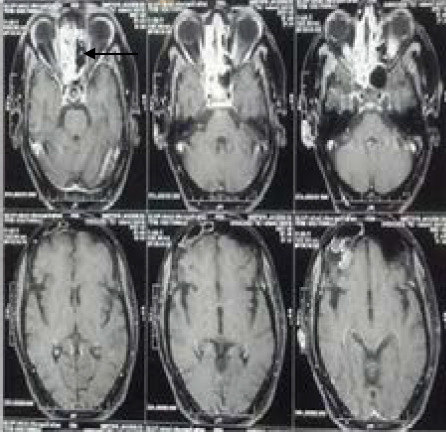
T1 Post-contrast MRI showing nonenhancing tissue in the left ethmoid sinus giving the Black turbinate sign. No intracranial and intraorbital extension noted.
